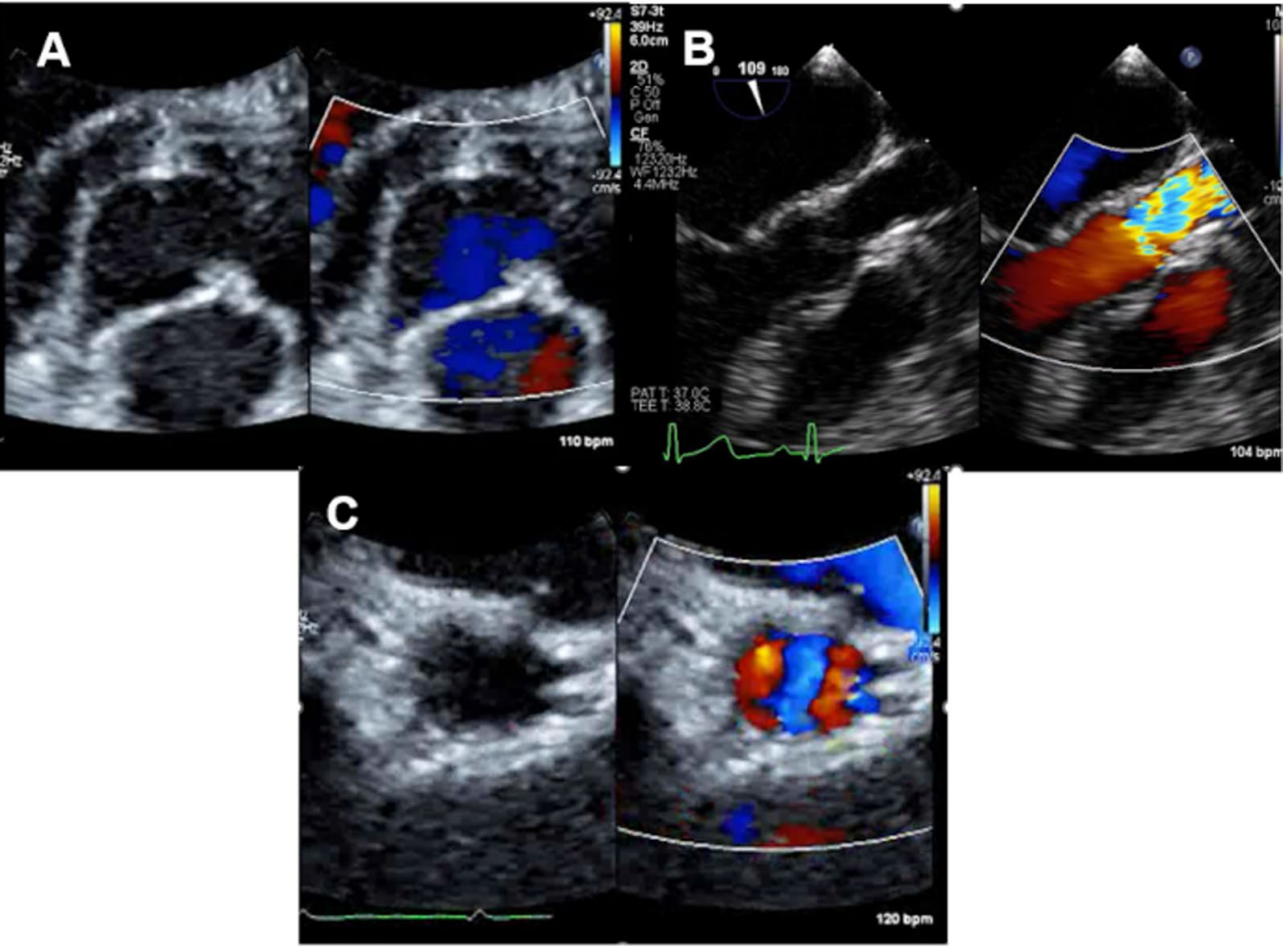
Echocardiographic findings of the aortic valve and the coronary arteries. A Echocardiography revealed partial coaptation of the non-coronary cusp (NCC) and right coronary cusp (RCC), resulting in a bicuspid aortic valve. B Aortic annulus was 10.3 mm, and the aortic stenosis was 8.0 mm. C Both coronary orifices of the left anterior descending artery and the left circumflex artery were located separately in the LCC
